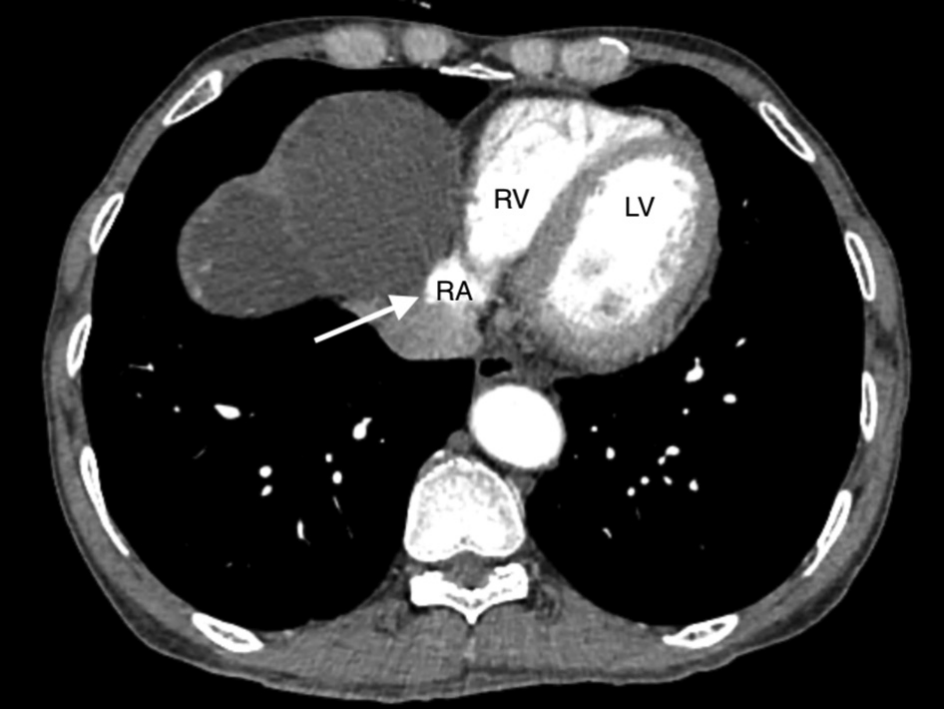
Right atrial compression by multiple massive hepatic cysts on computed tomography (*arrow*).
